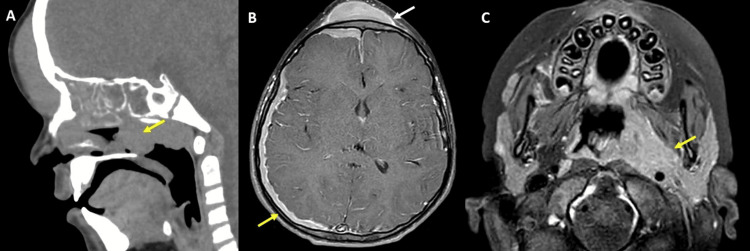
(A) Sagittal plain neck CT scan showing a solid frontal mass and thickening of the nasopharyngeal soft tissues (yellow arrow). (B) Contrast-enhanced axial brain MRI showing a solid mass with homogeneous enhancement located in the frontal epicranial soft tissue (white arrow), associated with pachymeningenal thickening and enhancement of the right cerebral hemisphere (yellow arrow). (C) Solid, multi-space-occupying mass showing homogeneous enhancement involving the nasopharynx, parapharyngeal space, carotid, and masticatory space on the left side (yellow arrow).
